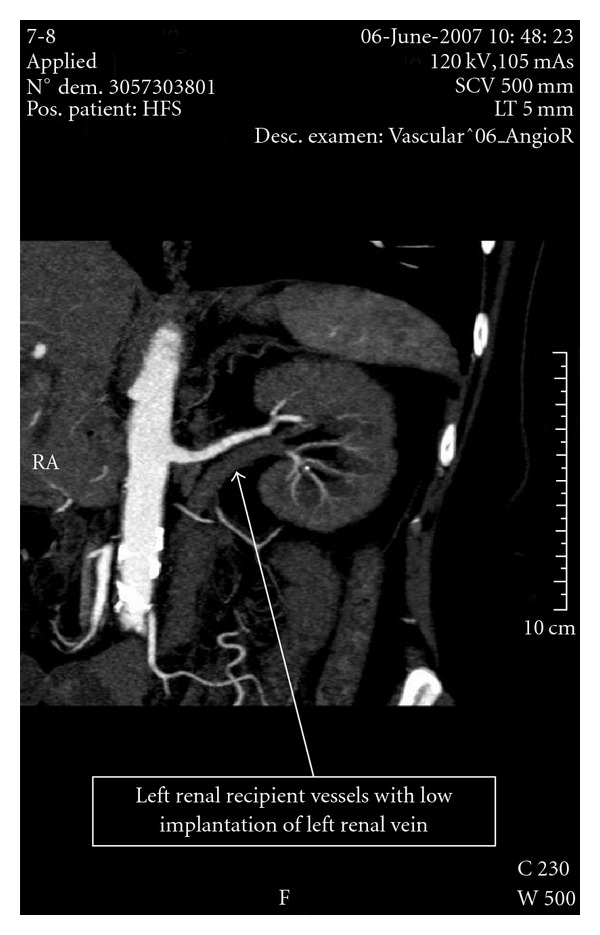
CT angiography image of the receiver left renal vessels.
